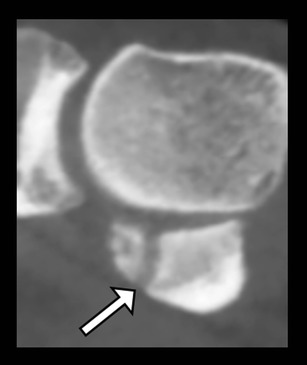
Bifid medial sesamoid bone of the hallux. Sagittal reformatted CBCT demonstrating the presence of this anatomical variant (arrow)
